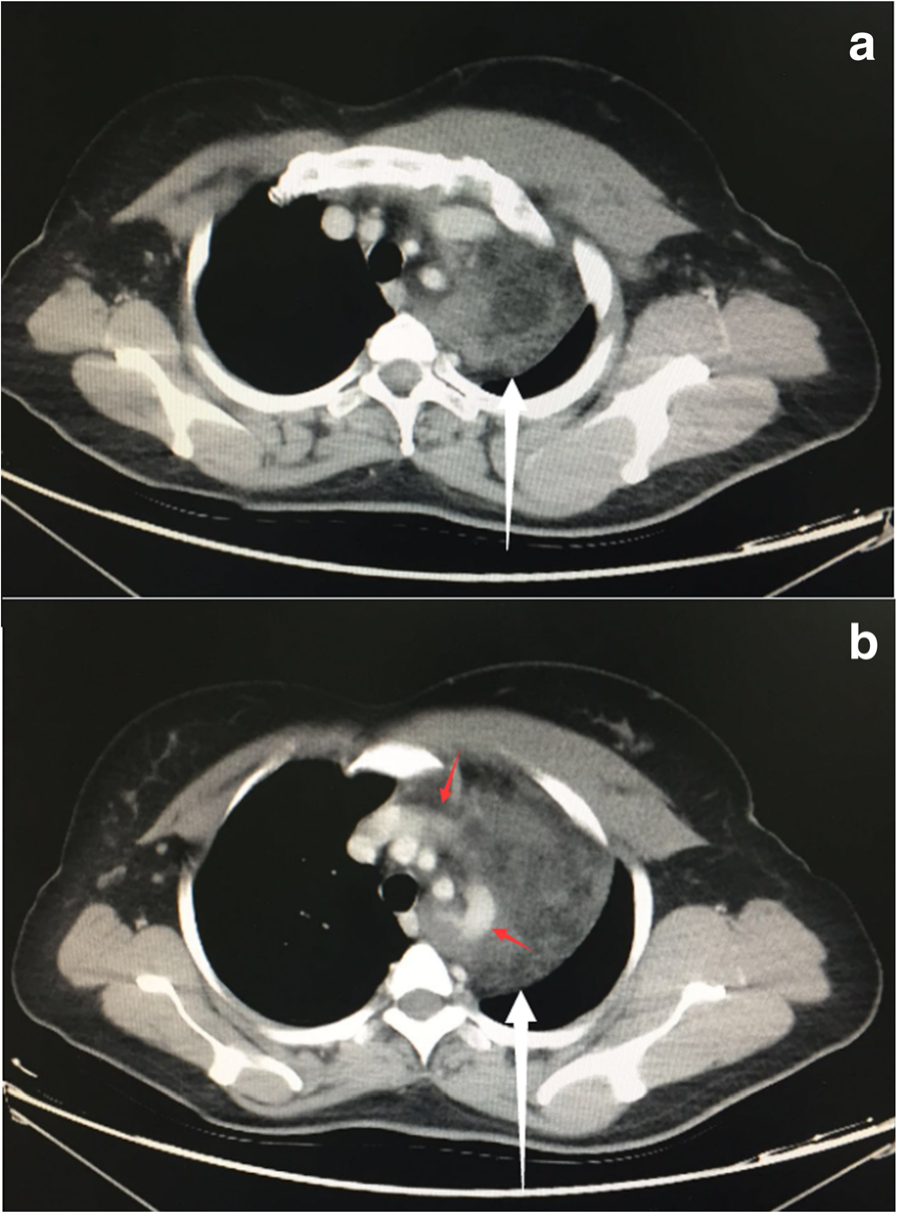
a CT scan of chest showed a 10*5.5 cm-sized mass (*white arrow*) with fat densities in the middle superior mediastinum. b The tumor was slightly heterogeneously enhanced and the adjacent blood vessel was surrounded by the lesion with obscure boundary(*red arrow*). The left chest and back muscles were thicker than the contralateral ones
